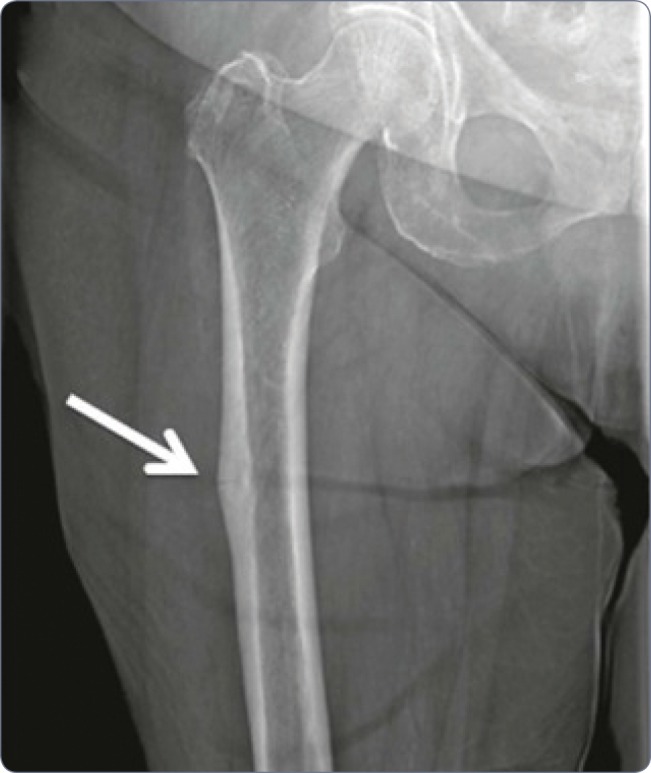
Radiograph of the right proximal femur showing an atypical, incomplete femoral diaphysis fracture (arrow) after long-term bisphosphonate therapy. Image adapted from Link and Adams (2016).
